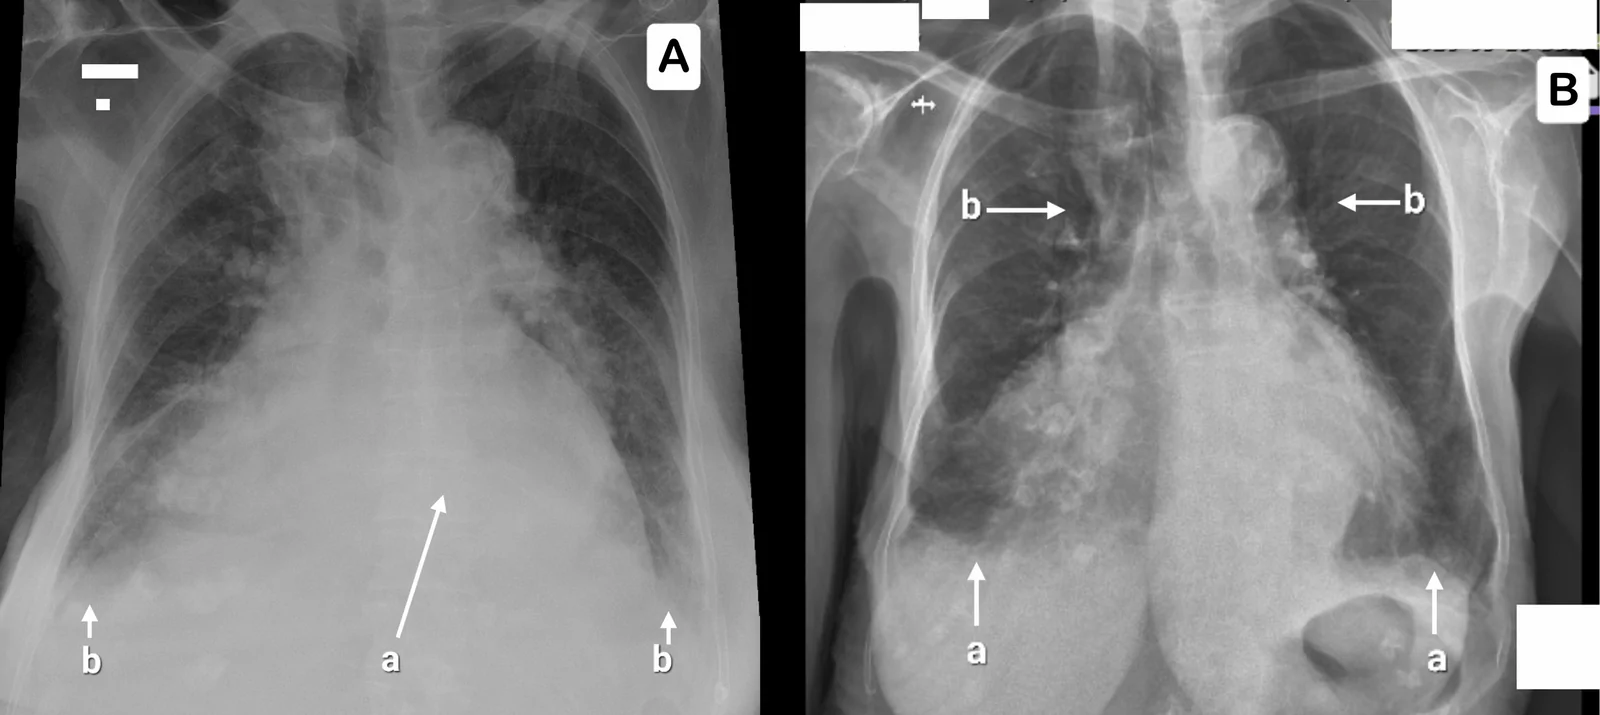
Chest radiographs before and during transfusion-associated dyspnea (A) Supine anteroposterior radiograph obtained on Day 1 after dyspnea developed during the second packed red-cell transfusion, showing marked cardiomegaly (arrow a), bilateral pleural effusions (arrows b), and pulmonary vascular congestion. (B) Upright posteroanterior radiograph obtained at emergency presentation on Day 0, showing cardiomegaly, small bilateral pleural effusions (arrows a), and perihilar opacities (arrows b); the formal radiology report stated that pulmonary venous congestion was absent. The panels are not directly comparable because they were obtained using different patient positions and acquisition techniques.
